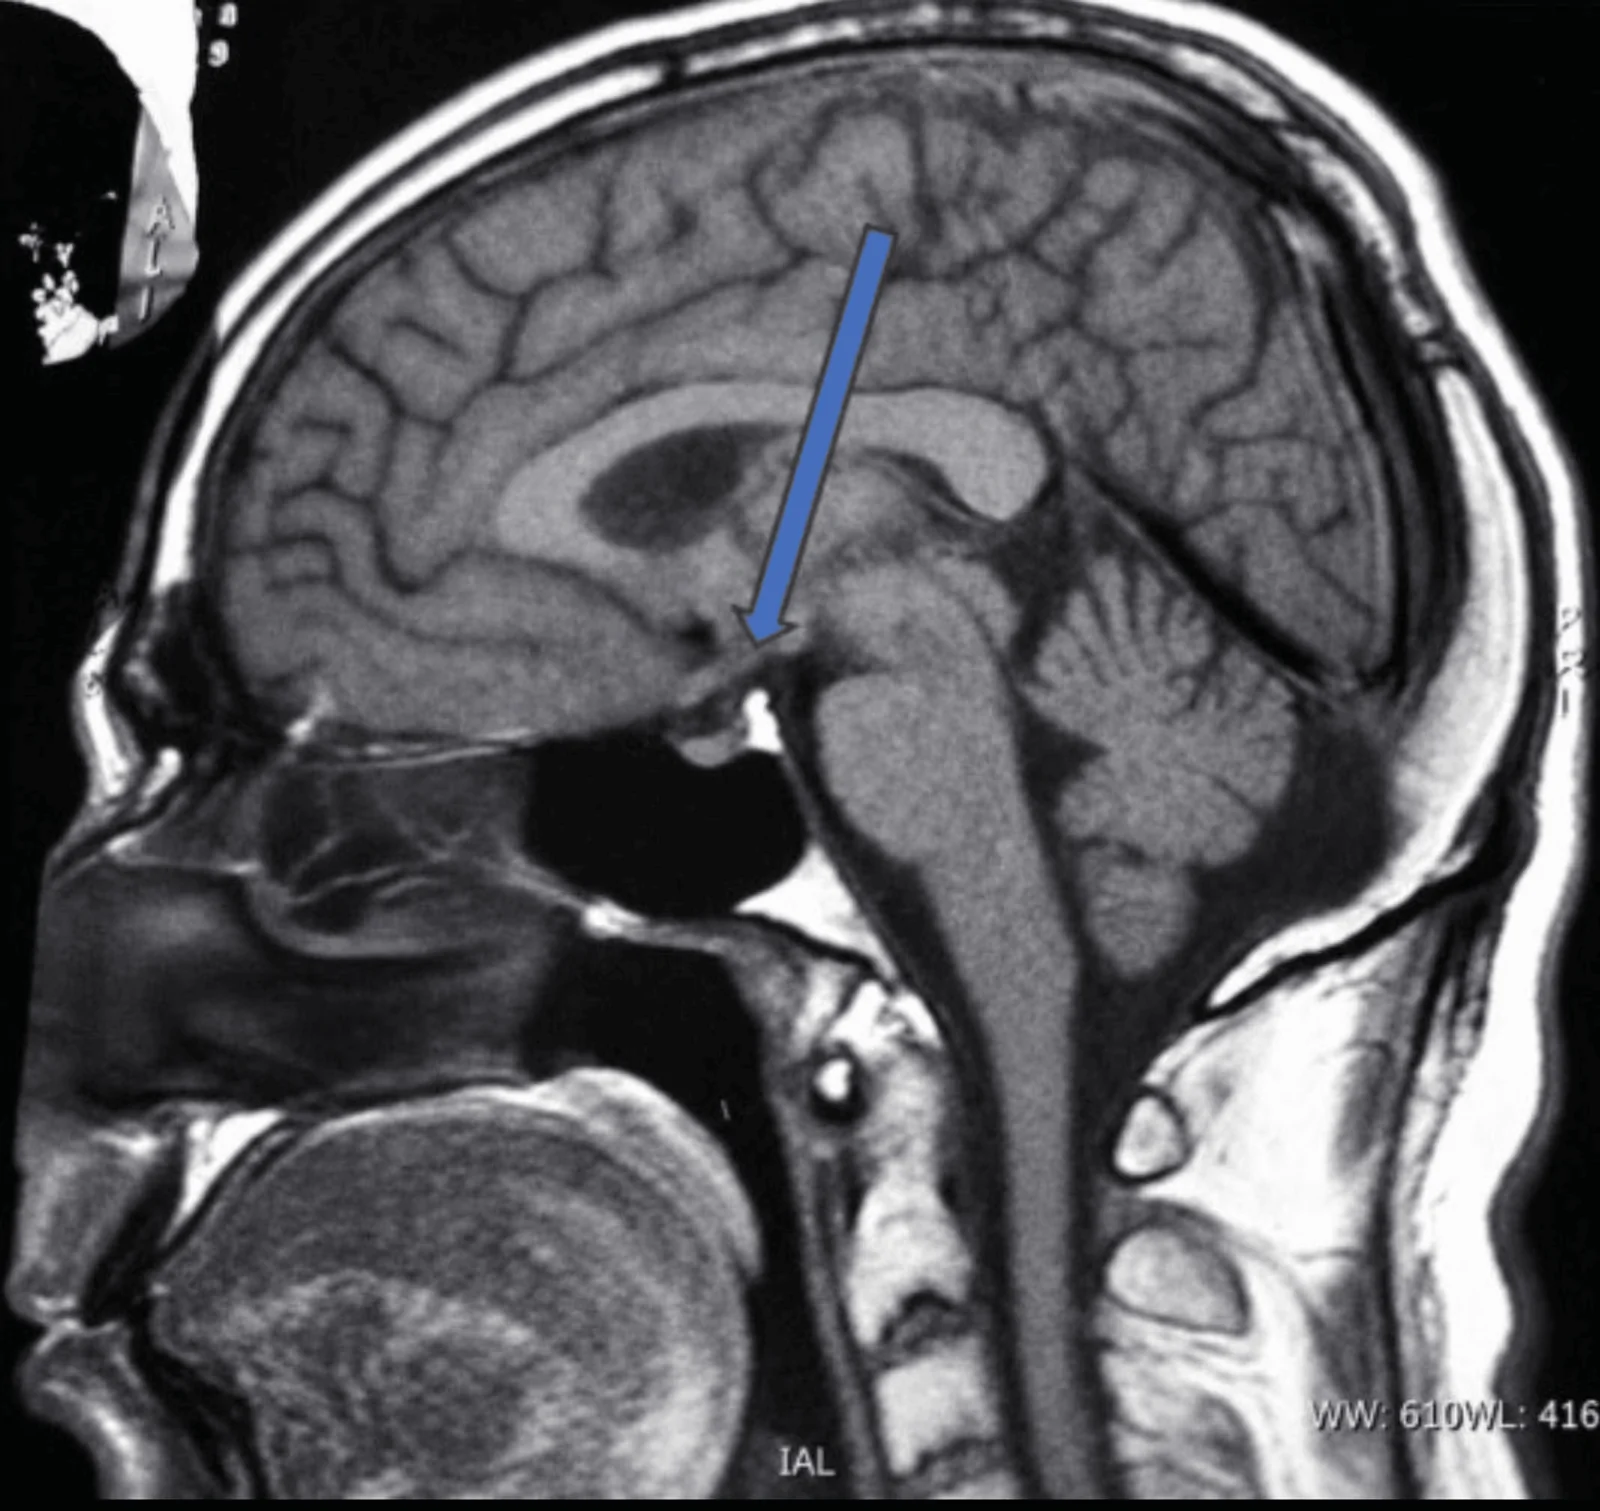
Sagittal T1-weighted MRI of the sella showing absence of the normal posterior pituitary bright spot (arrow), consistent with central diabetes insipidus. MRI: magnetic resonance imaging
